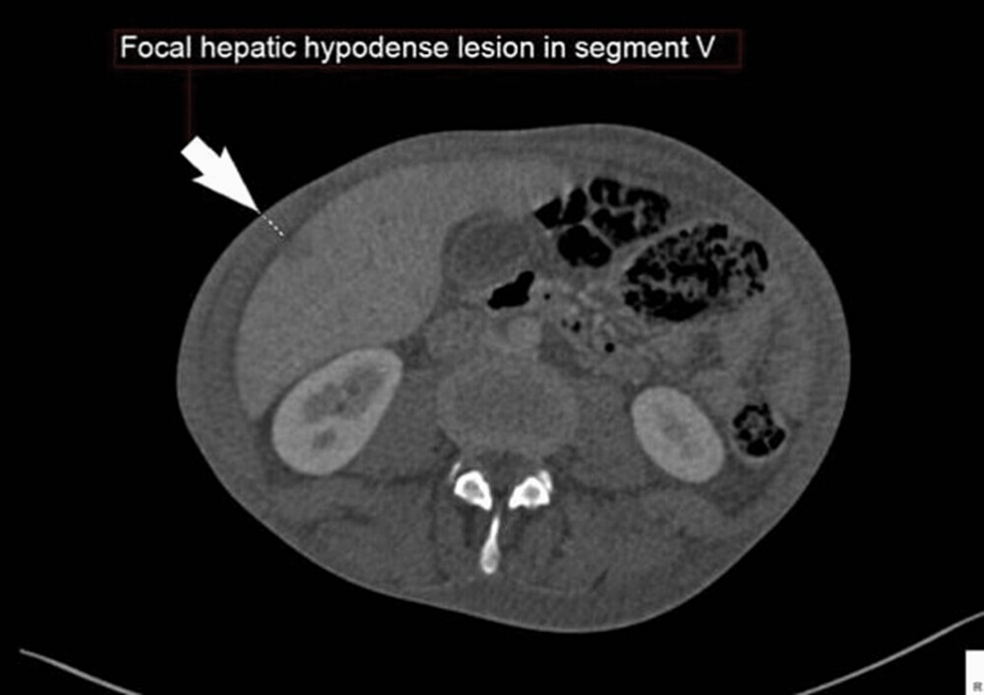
In this axial view of an upper abdominal CT scan, a metastatic lesion in segment V of the liver is clearly visible (arrow). CT, computed tomography
